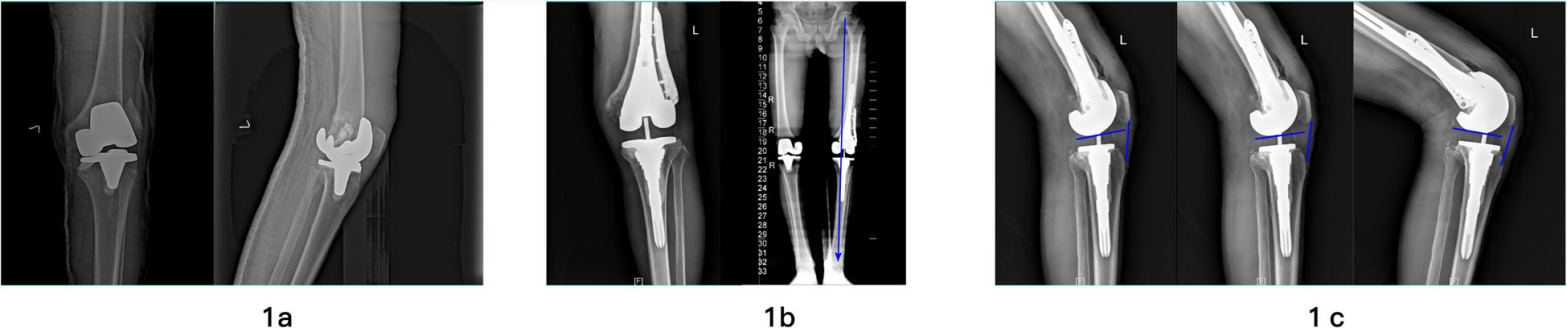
a Radiographs of a 68-year-old male who underwent revision TKA for aseptic femoral loosening and distal femoral fractures associated with an AORI type 3 femoral defect. b Postoperative full-length standing anteroposterior radiographs demonstrated the the knee was well-aligned and prosthesis was well-fixed. c Lateral views of the knee revealed no patella baja
